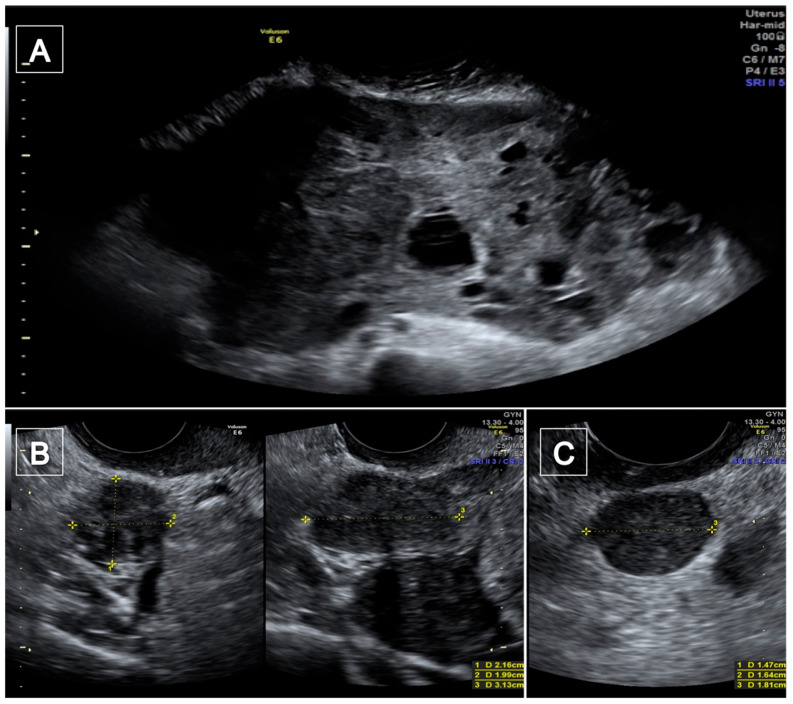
The trans-abdominal ultrasound examination. A voluminous mass with irregular margins and a heterogeneous echotexture for the presence of some cystic areas with anechoic content (A); two masses behind the uterus with regular margins, inhomogeneous echotexture and with acoustic shadows (B); a lesion of 14 × 16 × 18 mm with regular margins (C).
